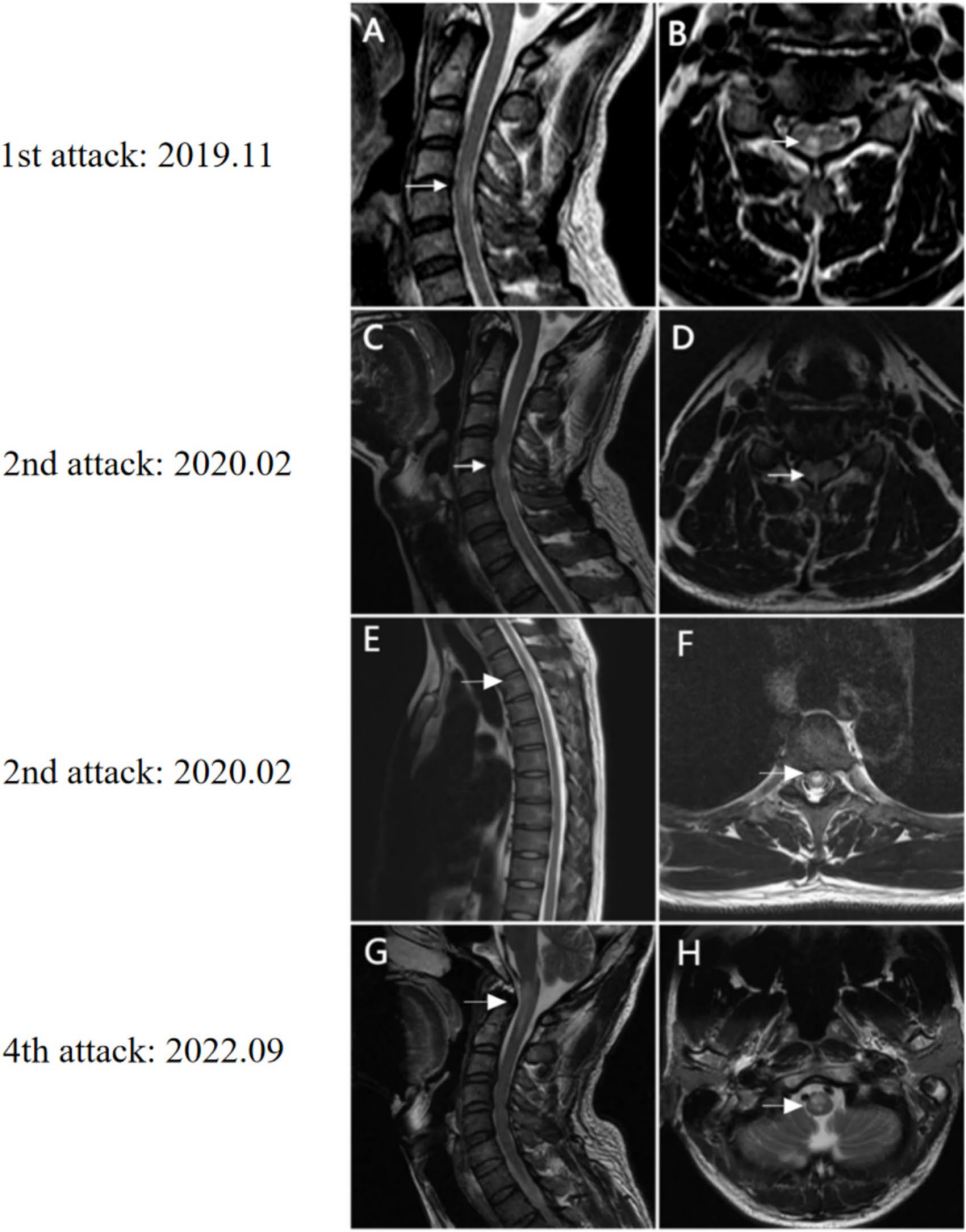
MRI findings of Case 1. (A) Sagittal T2-weighted cervical spinal cord MRI at first presentation shows a short-segment hyperintense lesion at the C4–C5 level (arrow). (B) Axial image demonstrating posterior spinal cord involvement (arrow). During the second attack, sagittal T2-weighted image (C) reveals C4–C5 hyperintensity (arrow), while axial imaging (D) shows posterior cord parenchymal involvement (arrow). Sagittal (E) and axial (F) T2-weighted images of the thoracic spinal cord demonstrate a new lesion at T4–T6 (arrows). Sagittal T2-weighted image (G) demonstrates a hyperintense lesion extending from the medulla oblongata to C2 (arrow), with (H) axial view confirming medullary involvement (arrow).
